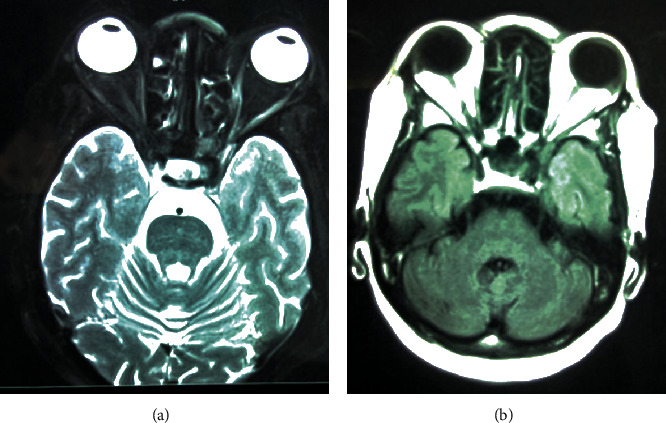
MRI axial slice of the brain in (a) T2 sequence and (b) T2-FLAIR sequence after contrast injection showing no abnormalities in the cavernous sinus.
